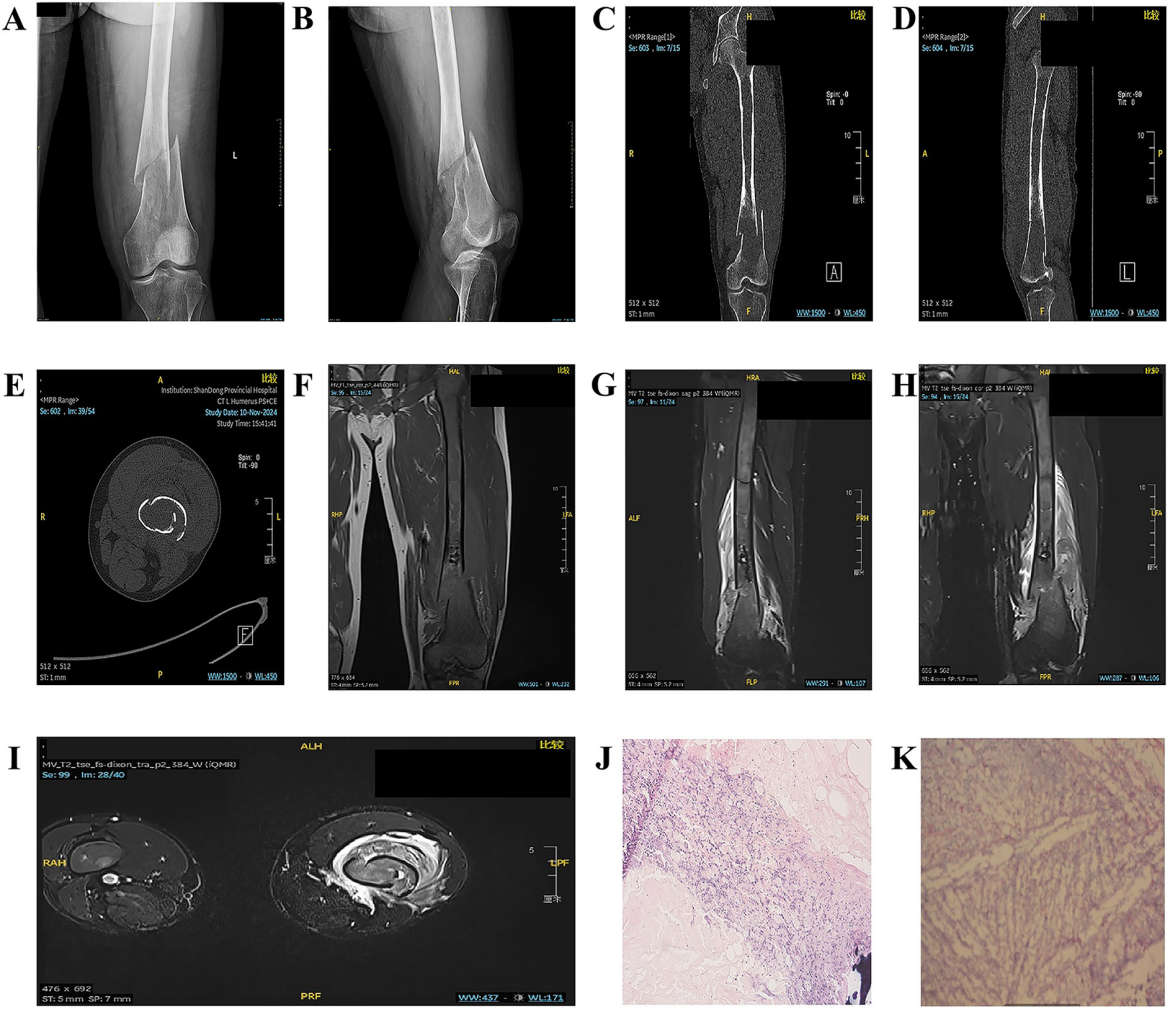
(A,B) Anteroposterior and lateral views of the left femur on DR. (C–E) Noncontrast 3D-CT scan of the left femur. (F–I) Noncontrast MR scan of the left thigh. (J,K) Observation results of paraffin-embedded sections of GD tissue.
